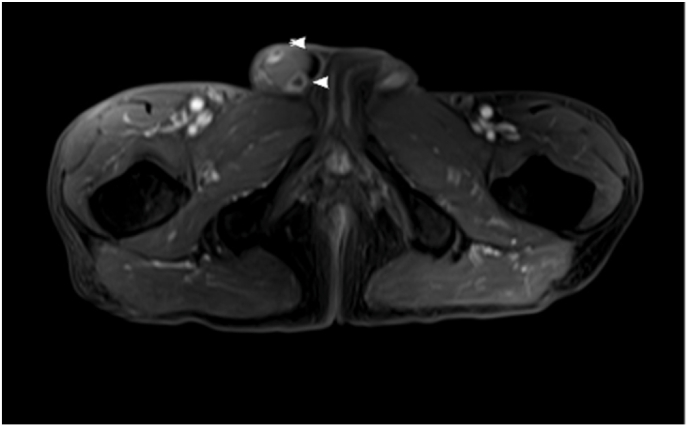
MRI identified right inguinal testis with adjacent rim enhancing nodules (arrowheads).
